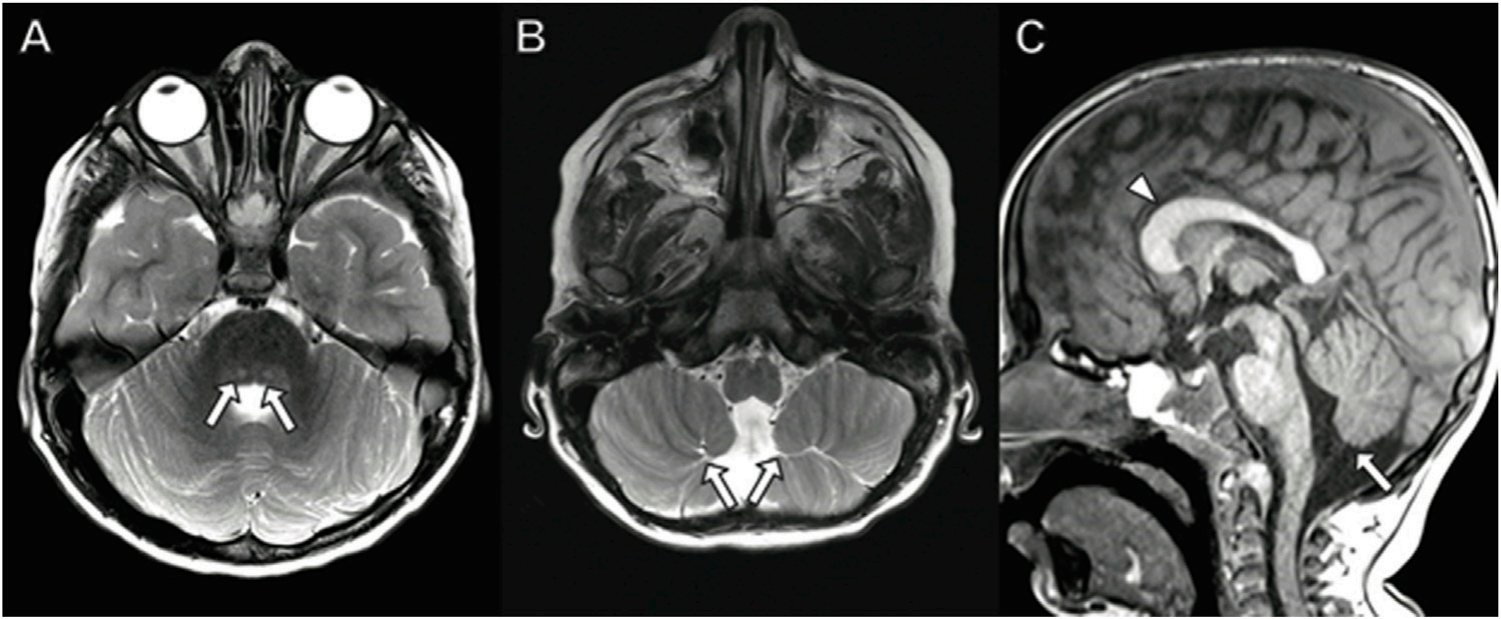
Brain MRI in a 3-year-old boy with *PACS2* mutation. (A) Axial T2 demonstrates hyperintense signal in the central tegmental tracts (arrows). (B) Axial T2 image shows dysplastic cerebellar folia (arrows). (C) Sagittal T1 image with dysplastic, abnormally thick genu of the corpus callosum (arrowhead) and vermian hypoplasia (arrow).
